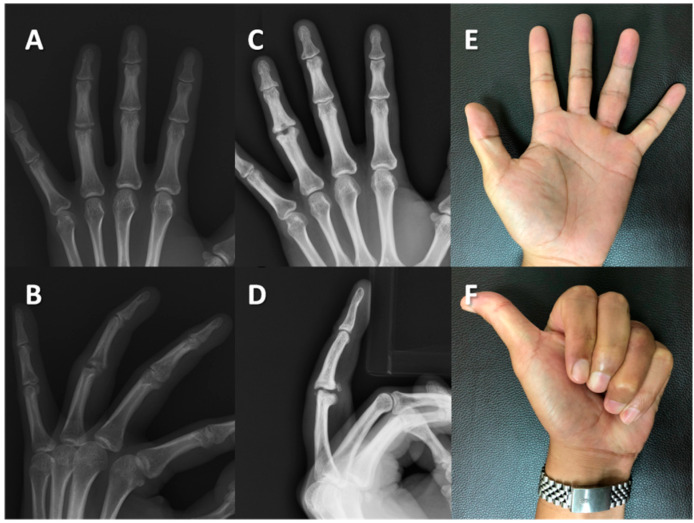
Case #5: A 33-year-old man developed post-traumatic osteoarthritis of the left ring finger. Radiographs of the left ring finger in the (A) anteroposterior (AP) view and (B) lateral view revealed post-traumatic osteoarthritis of the proximal interphalangeal joint in the preoperative status. The six-year follow-up assessment revealed preserved joint space in the (C) AP and (D) lateral views. The active flexion of the finger was 90° (E) with no extensor lag (F).
